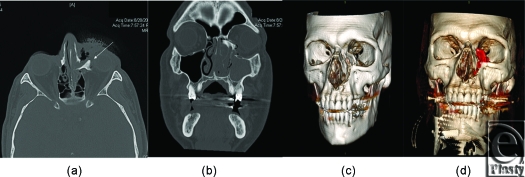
Computed tomography scan demonstrating injury pattern (a) axial view with unilateral nasal orbital ethmoid (NOE) segment rotated 180° into ectopic position, (b) coronal view demonstrating the ectopic bone from left nasal piriform rotated posteriorly, (c) 3D reconstruction of the bony injury left maxilla, and (d) red indicates the 22-mm segment of bone rotated posteriorly into the orbit. This ectopic bone was interposed between the inferior oblique and the medial rectus muscles of the left globe.
